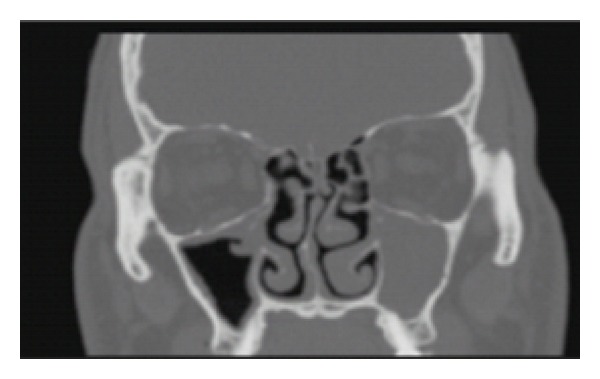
Computed tomography of the paranasal sinuses showing complete obstruction of left maxillary sinus and signs of chronic maxillary sinusitis on right side.
